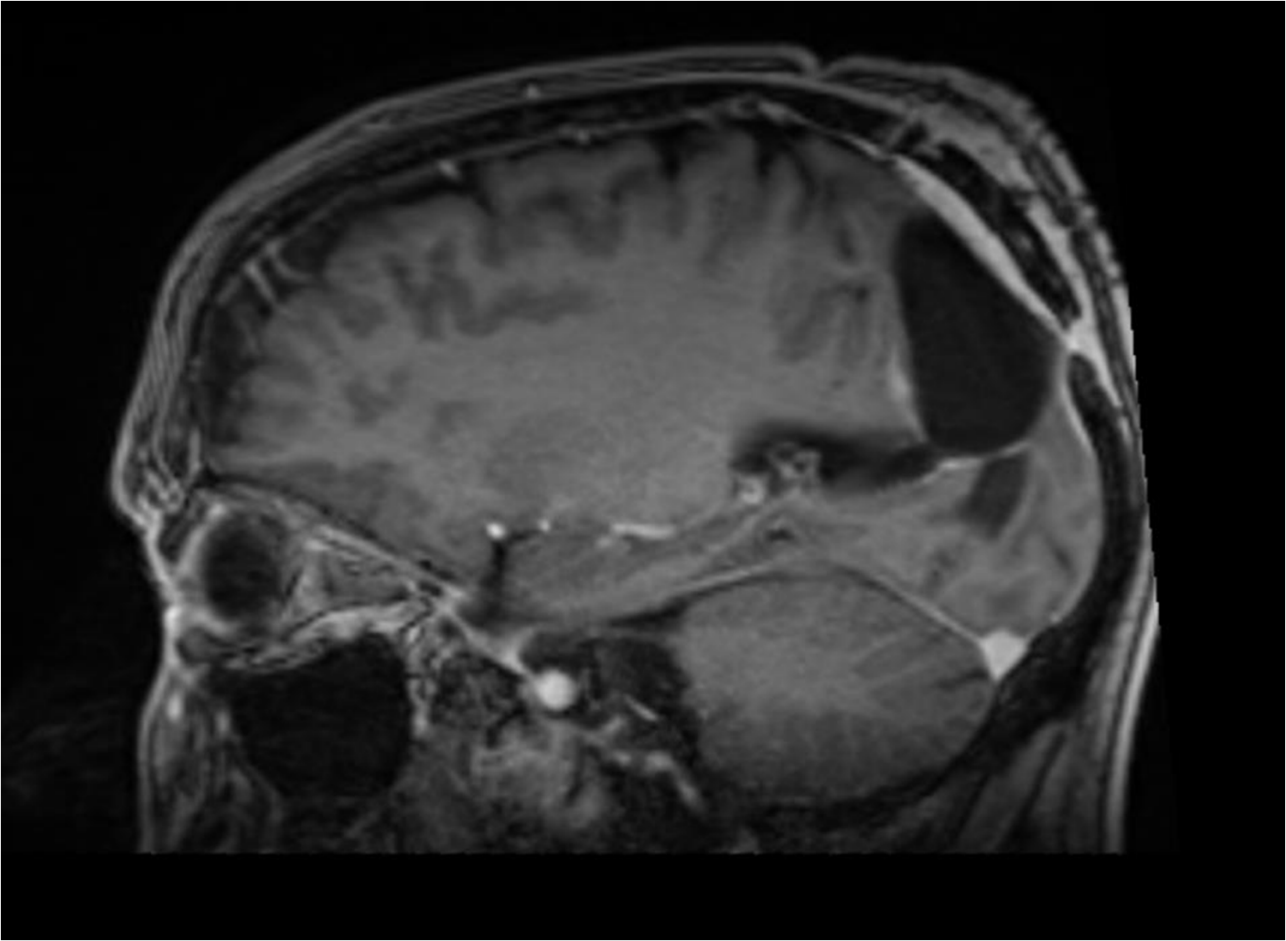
Postoperative sagittal T1-weighted MRI showing no residual subcutaneous CSF. Note the openly communicating tumor bed and posterior horn of the lateral ventricle
